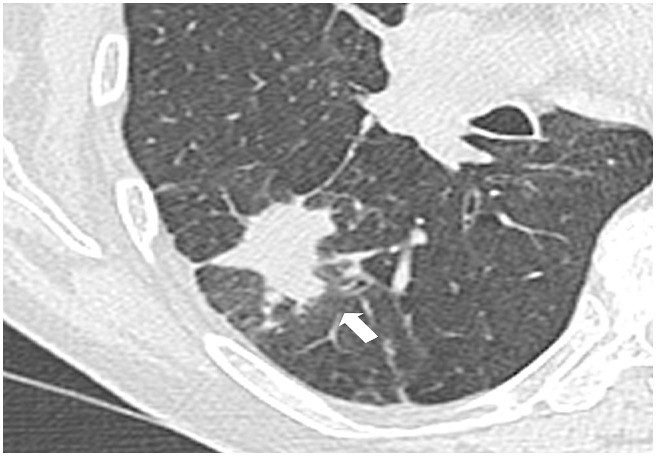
First unenhanced CT scan, with axial reformations, showing a 33-mm spiculated mass of the apical segment of the lower right lobe (large arrow)
